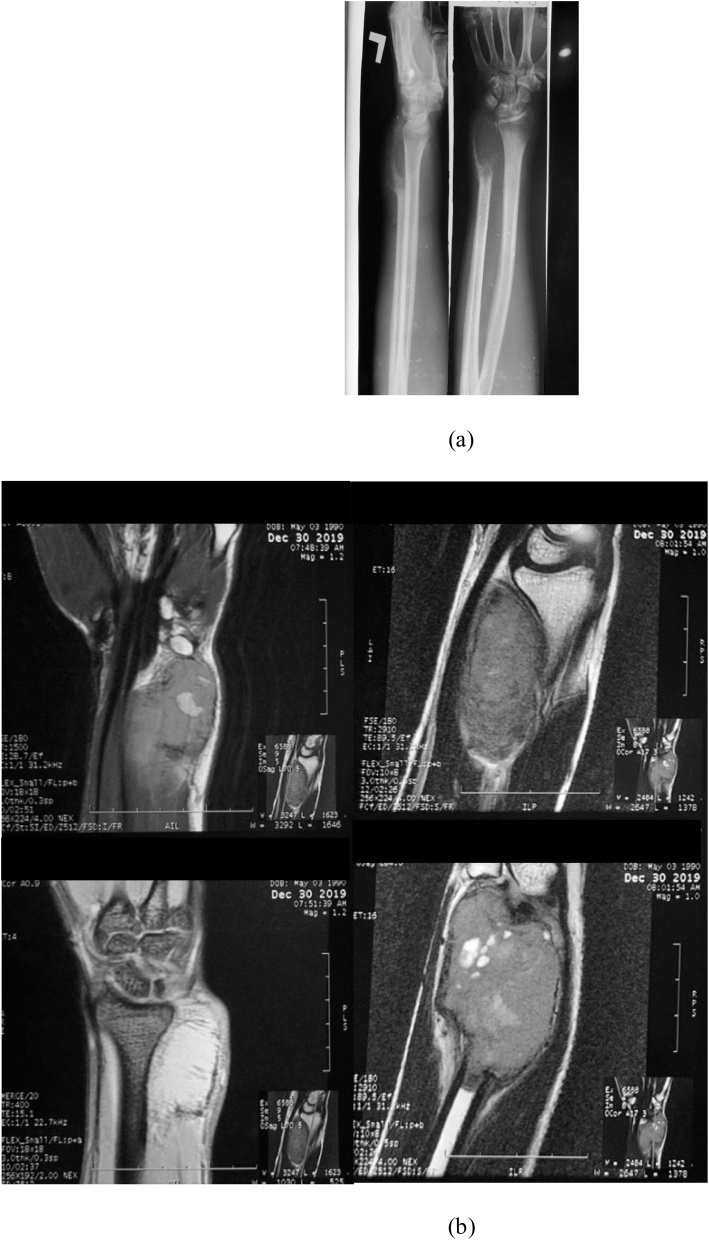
(a) Preoperative plain X-ray showed an expansile, multi-lobular, and radiolucent lesion with a clear margin in the distal end of the left ulna (b) MRI of the left wrist.
